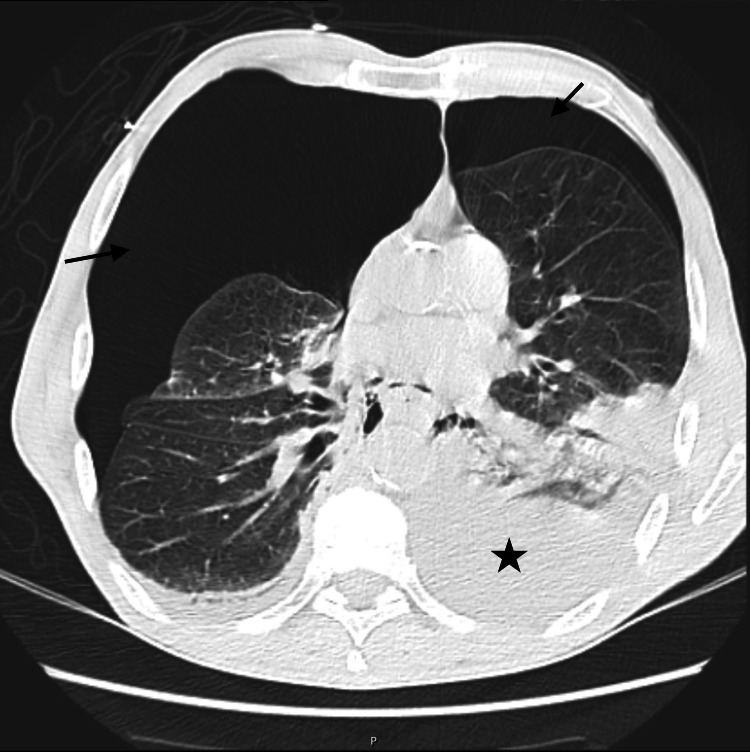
First CT scan Chest computed tomography (CT) revealed large, bilateral tension hydrothorax (star) and pneumothorax (arrow). Increased pleural effusion can be observed in the left hemithorax and atelectasis in the right medial lobe and both inferior lobes with reduction of the total lung volume predominantly on the right side.
